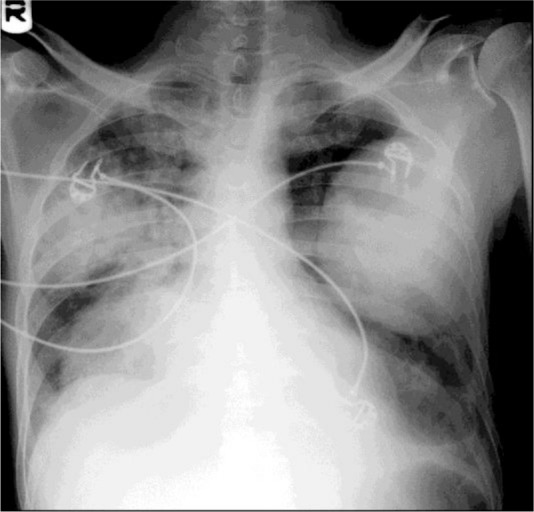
Chest X-ray shows severe pneumonitis with corresponding cysts.
